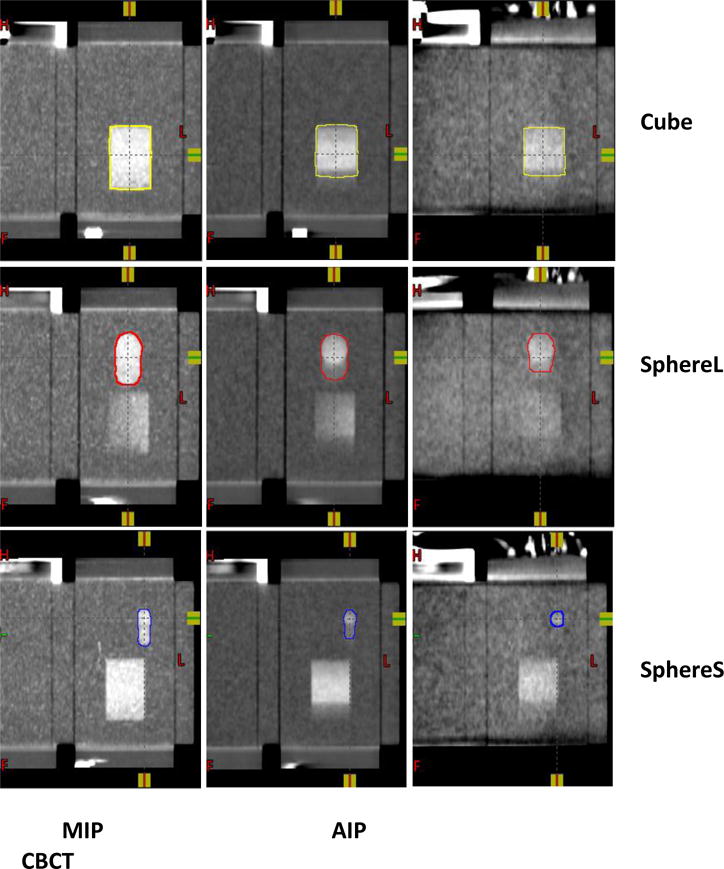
Targets contouring in MIP, AIP and CBCT images.
